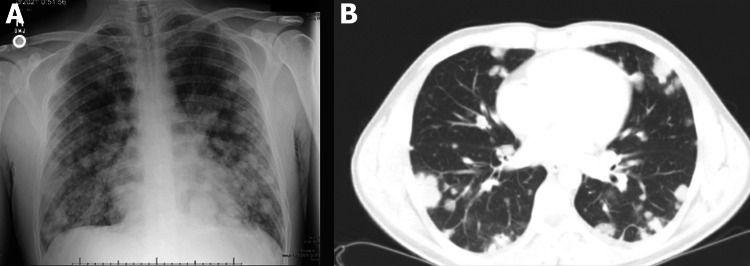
(A) Chest X-ray with diffuse pulmonary nodules and masses. (B) CT scan of diffuse pulmonary metastases. CT, computerized tomography
